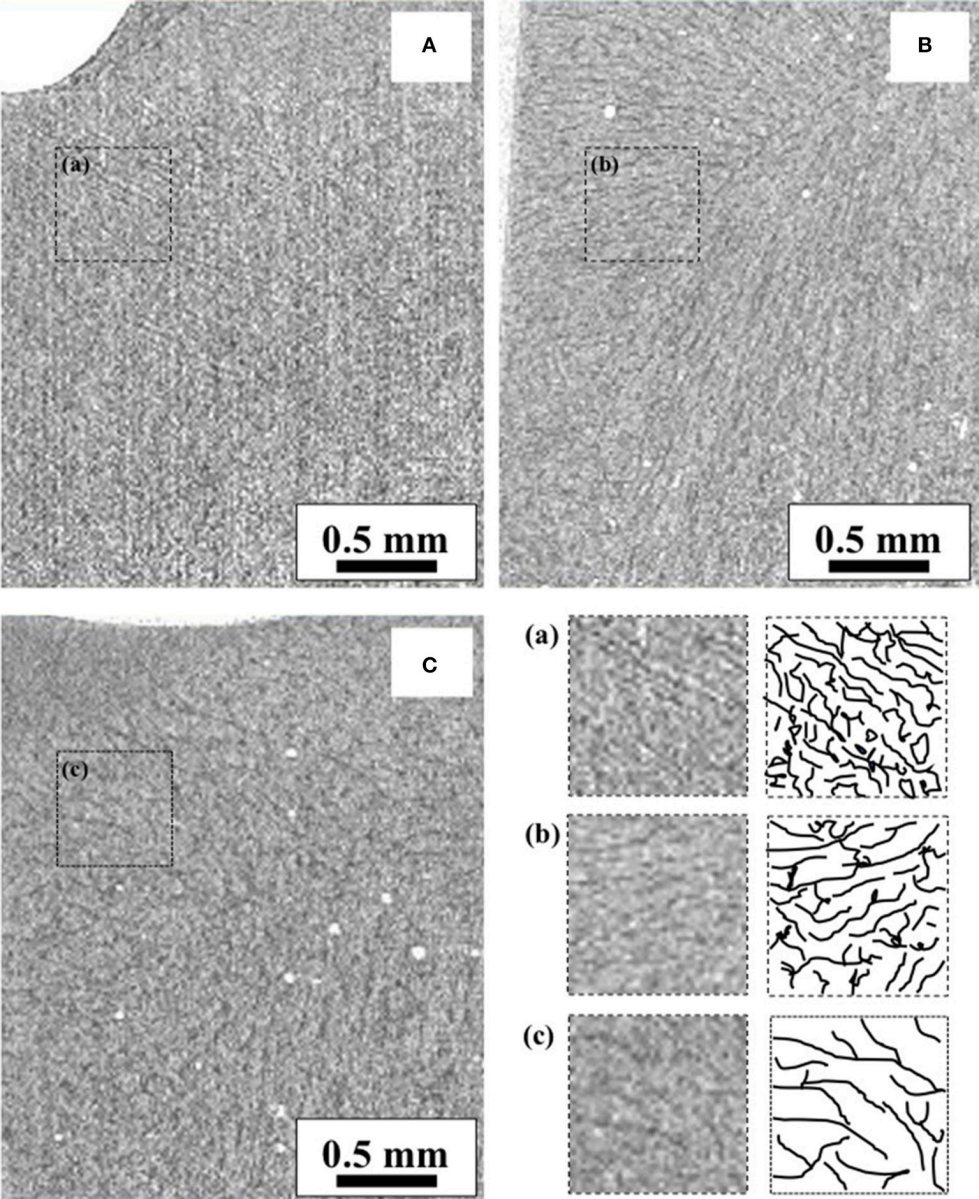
X-ray CT images of frozen samples: (A) non-annealed, (B) annealed for 12 h, and (C) annealed for 35 h.
